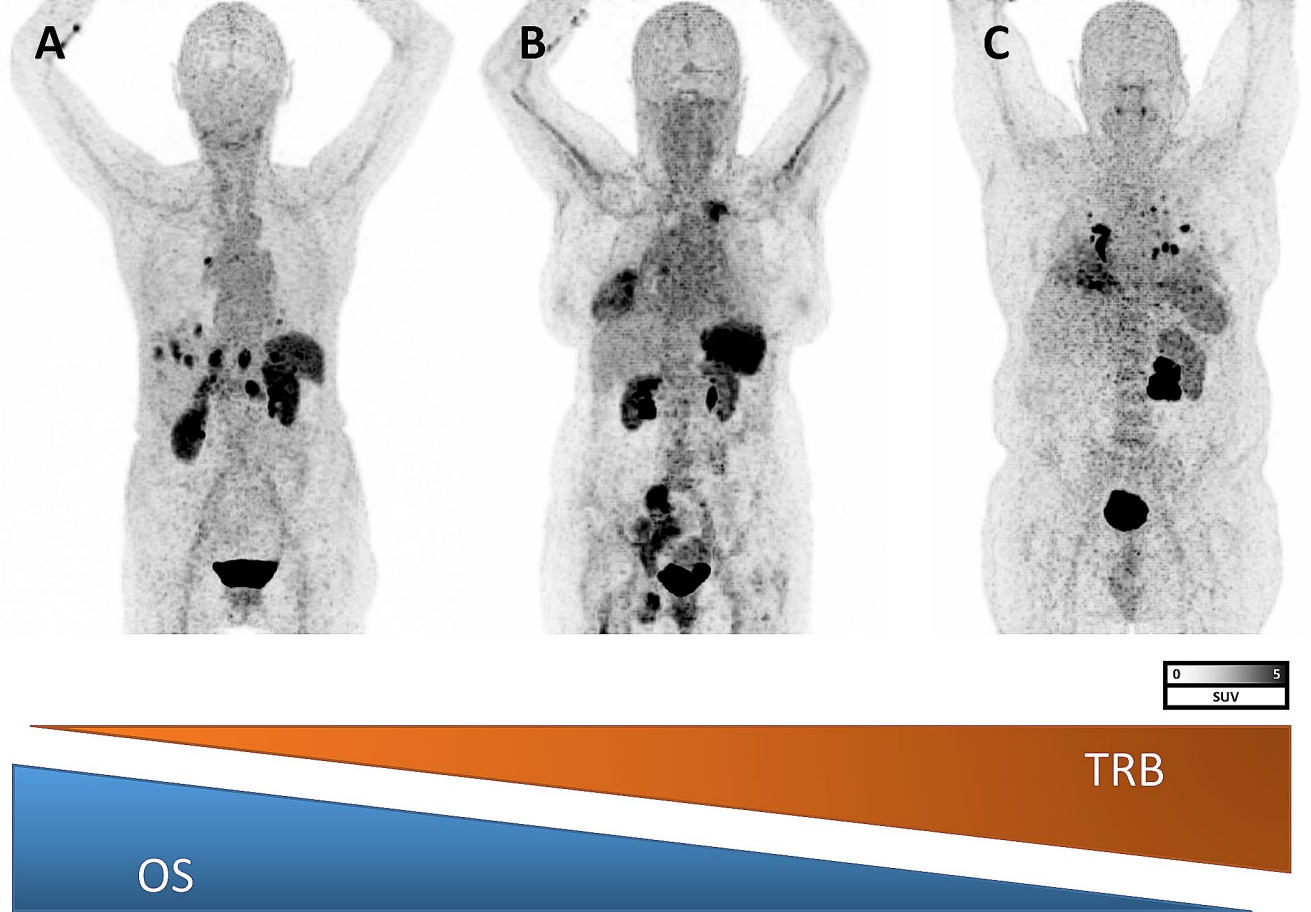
Example of three patients with [^68^Ga]Ga-PentixaFor PET/CT. Patient in A had a tumor receptor binding (TRB) of 259 and died 38 months after CXCR4-targeted molecular imaging, while Patient in B presented with TRB of 433 and survived 18 months. Patient C had the shortest overall survival (OS) with 9 months and the highest TRB of 645. Tumor volume, however, was virtually similar (A, 70 ml; B, 85 ml; C, 65 ml), thereby indicating that increased CXCR4 binding, but not PET-avid volume is of relevance for identifying high-risk patients
